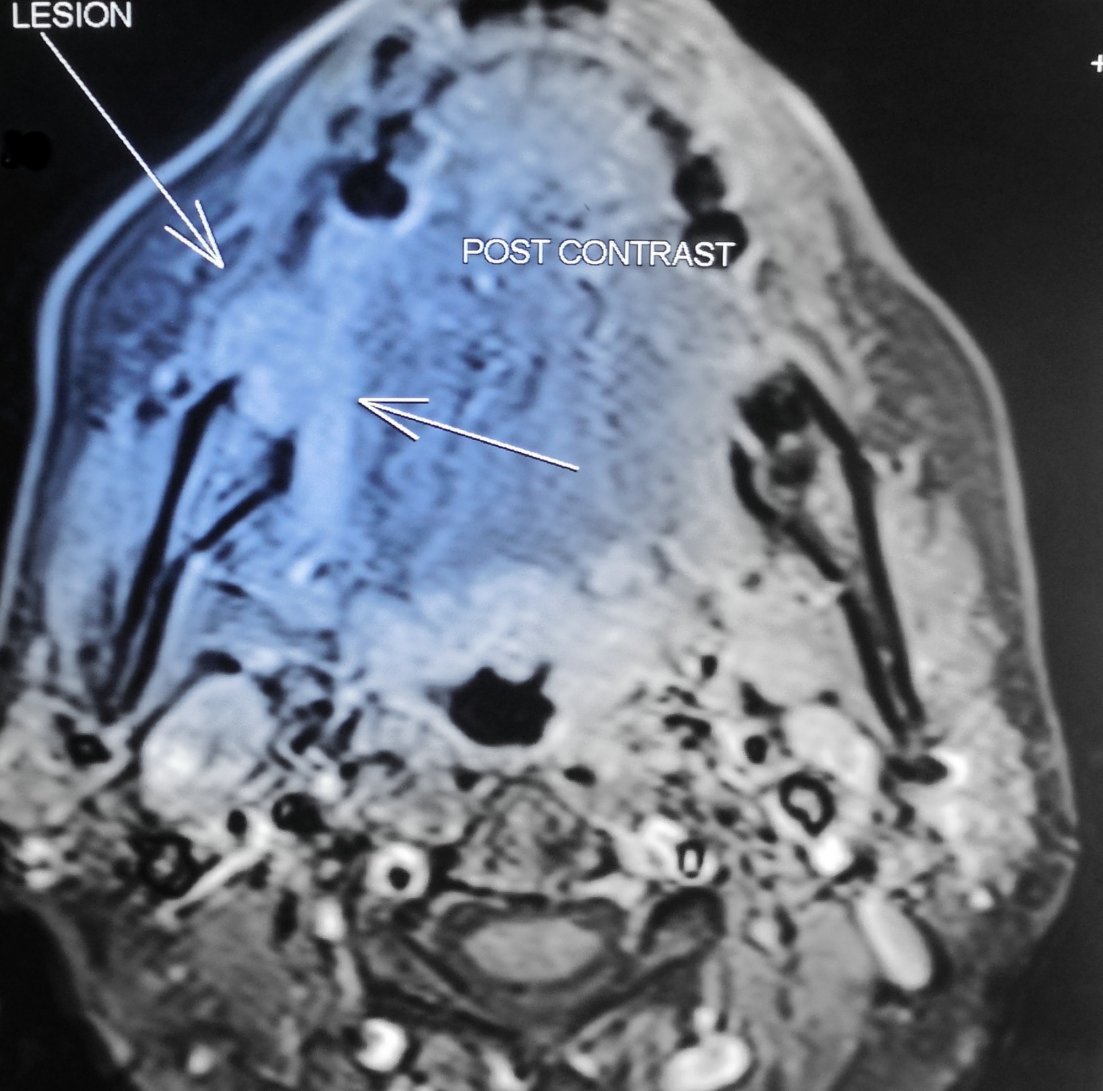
Contrast magnetic resonance image - axial section.
